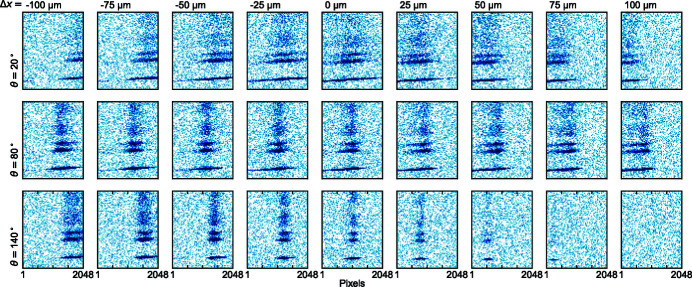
Panels showing the movement of the RIXS signal on an Andor CCD detector for different X-ray incident angles (θ) and sample *x* positions. The top, middle and bottom rows correspond to θ = 20°, 80° and 140°, respectively. The panels in each column correspond to increments of *x* in steps of 25 µm in negative or positive directions from the central position.
